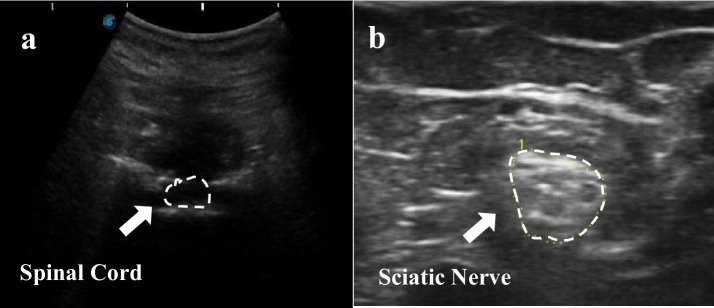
Ultrasonic localization of the spinal cord and sciatic nerve. (a. Spinal Cord; b. Sciatic Nerve).
